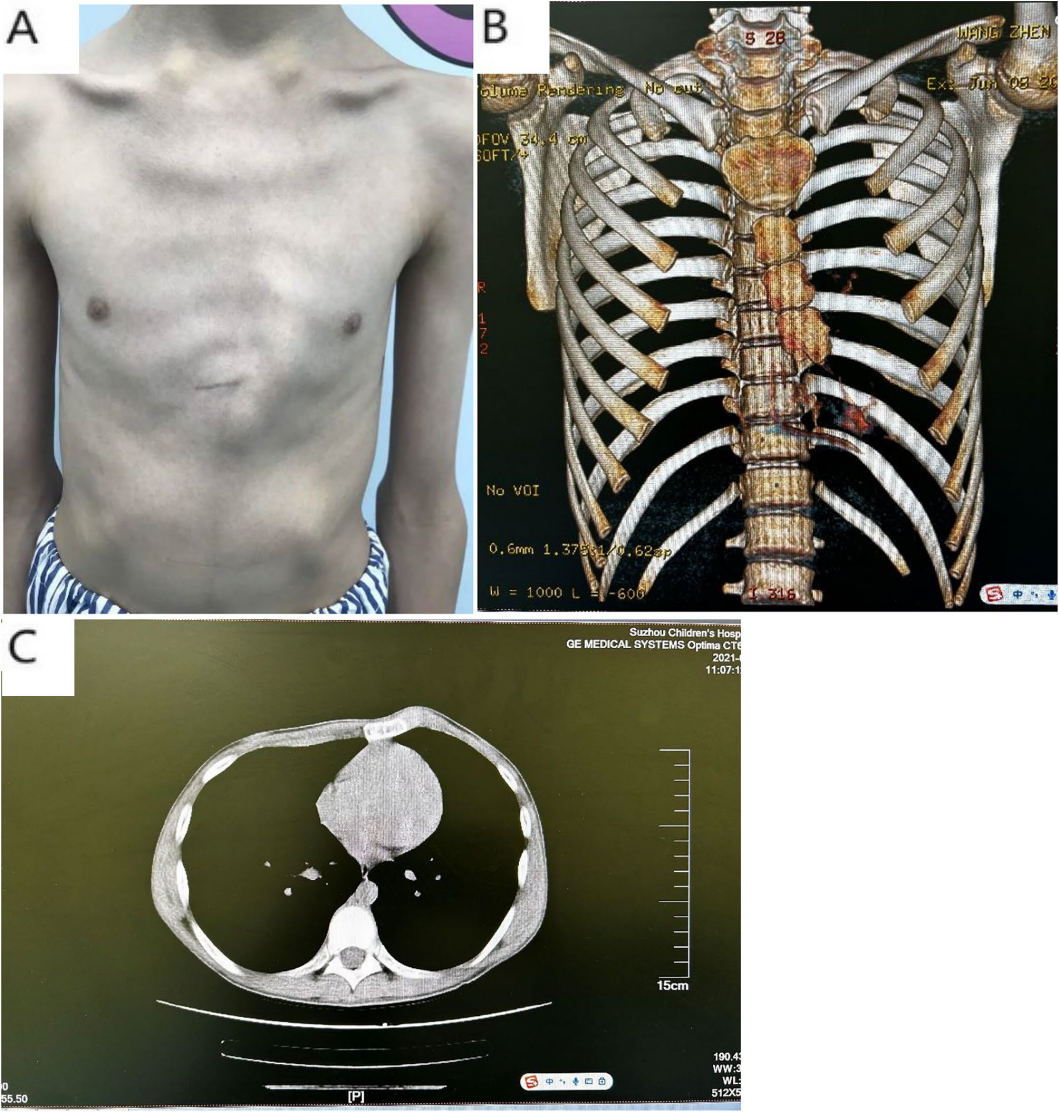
Chest appearance (a)and CT scan(b, c) of asymmetrical PC.B and C show the tilted and rotated sternum
